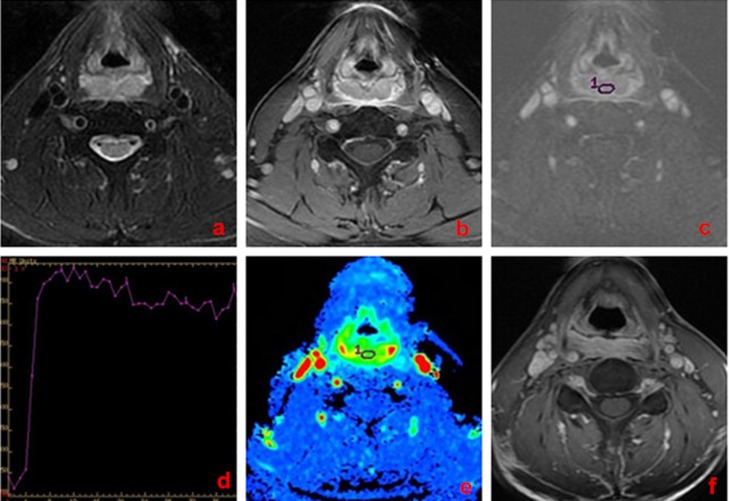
A 62-year-old man with hypopharynx carcinoma, poor response to chemo-radiotherapy a. Transverse T_2_-weighted imaging. b. Transverse T_1_-weighted enhancement imaging. c. DCE-MRI map. d. TIC map. e.The MSI map, MSI value was calculated as 65.72. f. Transverse T_1_-weighted enhancement imaging show that tumor mass was slightly reduced (< 30% reduction) after treatment.
